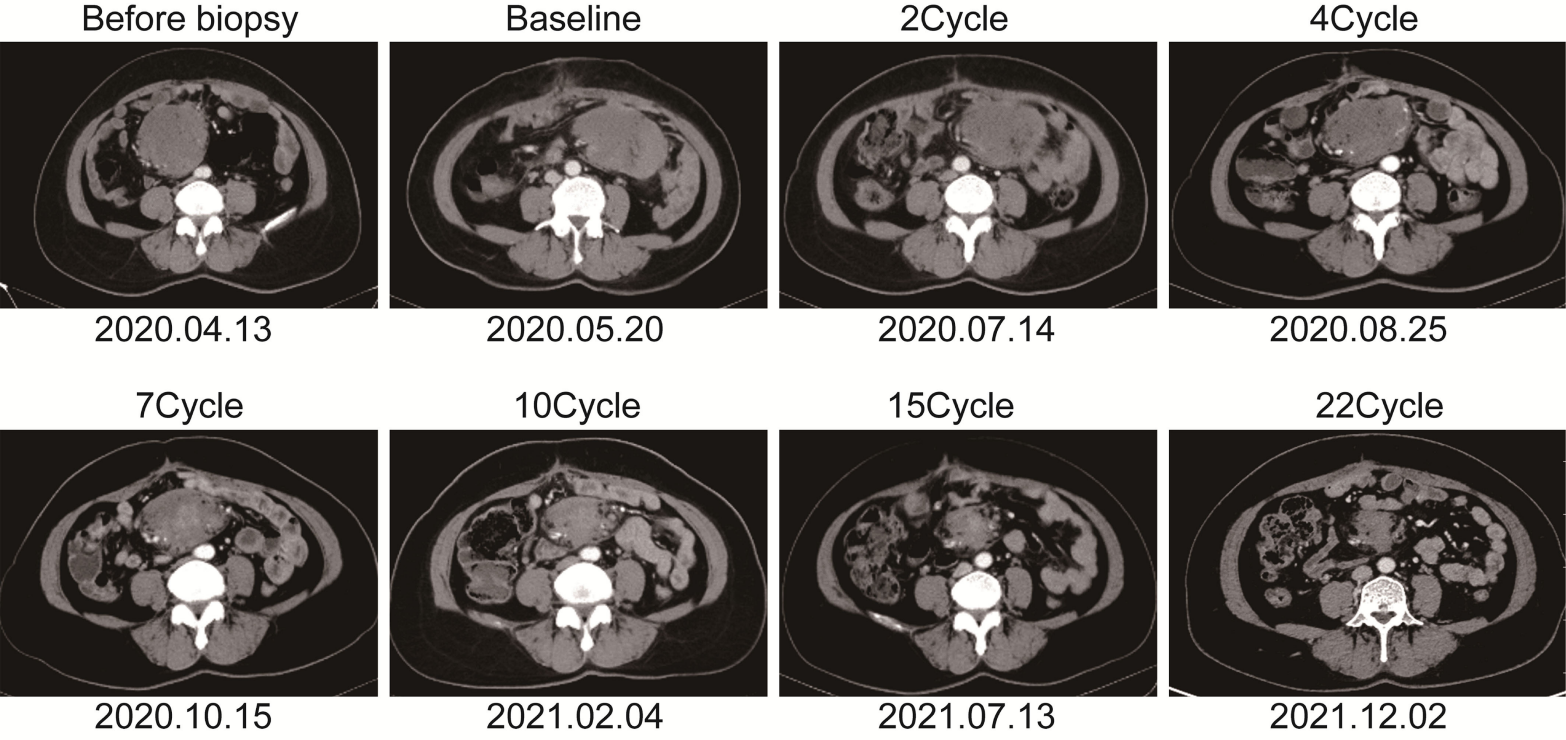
The soft tissue mass in the left lower abdomen was scanned with enhanced computed tomography at different time points.
